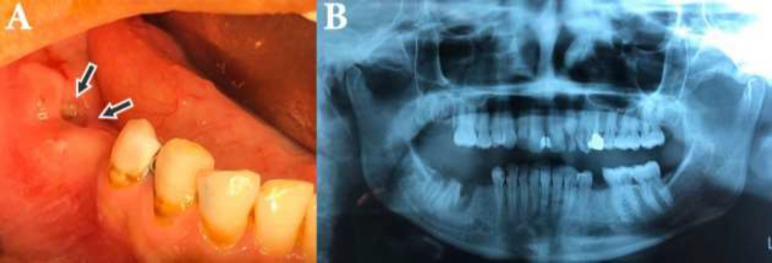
Clinical (a) and radiographic (b) view of MRONJ
